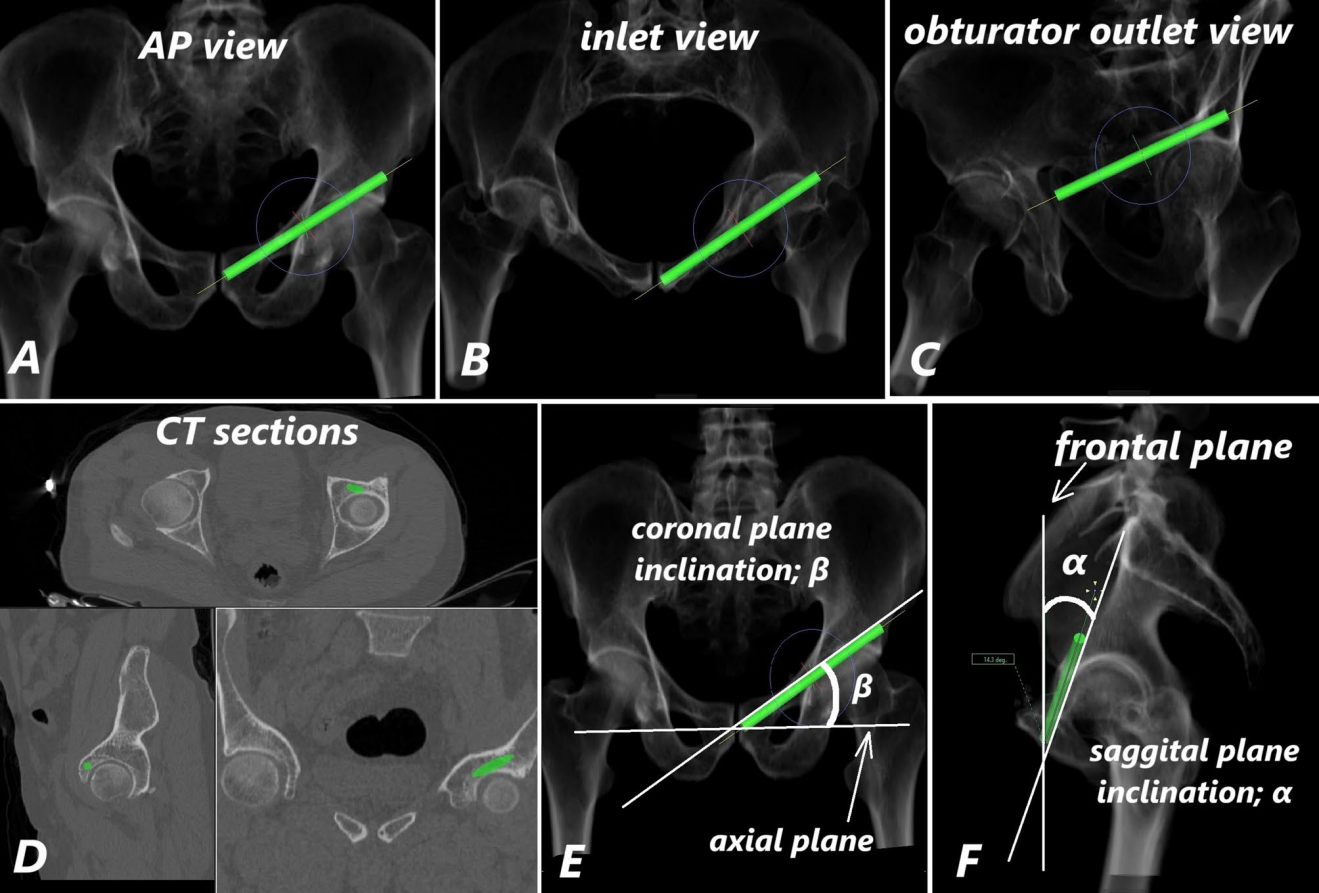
Fluoroscopic views (AP, inlet, obturator outlet) and axial, sagittal, coronal CT sections showing the screw within the ACFC without breaches (A-D). Measurement of fluoroscopic angles: CPI and SPI (E-F)
